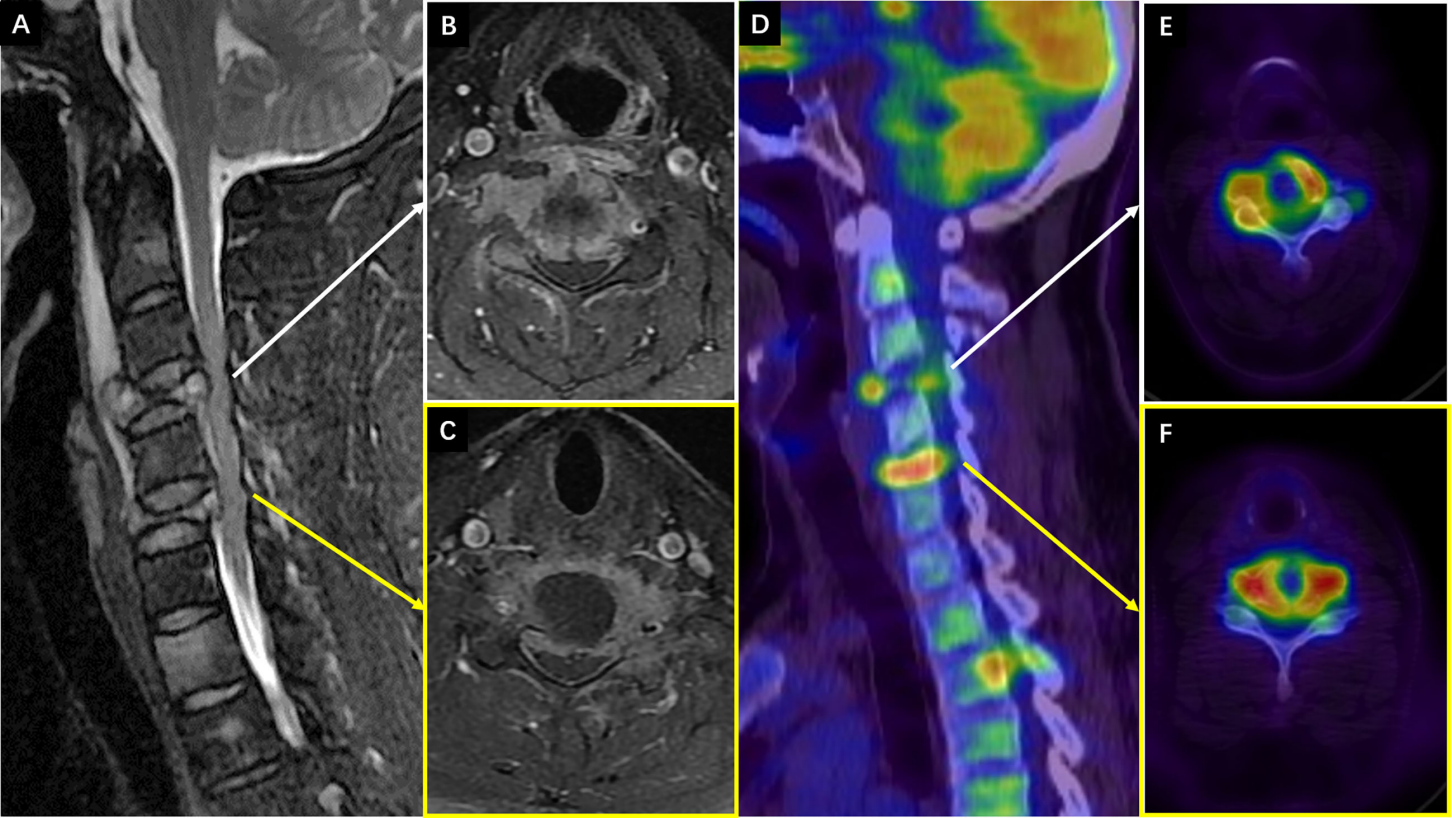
(A–C) The preoperative cervical spine MRI (8^th^ Dec. 2020). (A) Sagittal T2 weighted imaging showed vertebral collapse at C4 and C6 that compressed the spinal cord. (B, C) The axial T1 weighted imaging with contrast showed irregular enhancement of the metastatic lesions at C4 and C6 respectively, and compression of the spinal cord and vertebral arteries (right side at C4 and left side at C6). (D–F) The preoperative PET/CT images with views and levels corresponding to the MRI showed increased radioactive uptake at C4 and C6 (22^nd^ Dec.).
